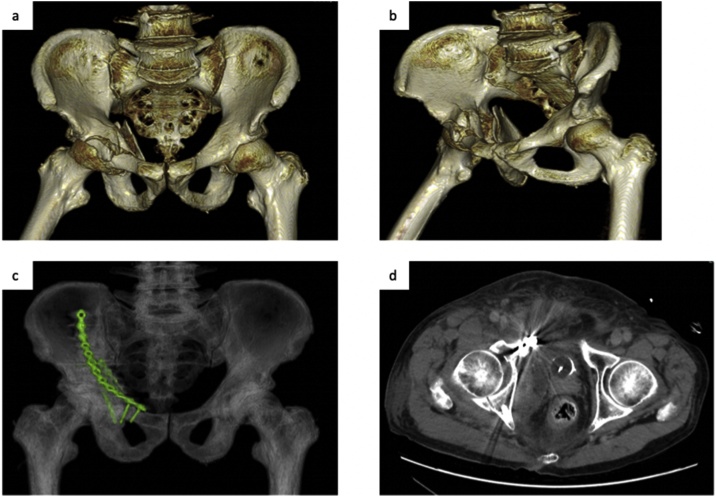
Pre- (a, b) and post-operative (c, d) computed tomography. a, b: Three-dimensional reconstructive computed tomography (CT) showing the right pelvic (acetabulum) fracture. Both anterior and posterior column are fractured. c: Open reduction and internal fixation (ORIF) was performed. d: CT showed retroperitoneal hematoma and no evidence of inguinal hernia.
